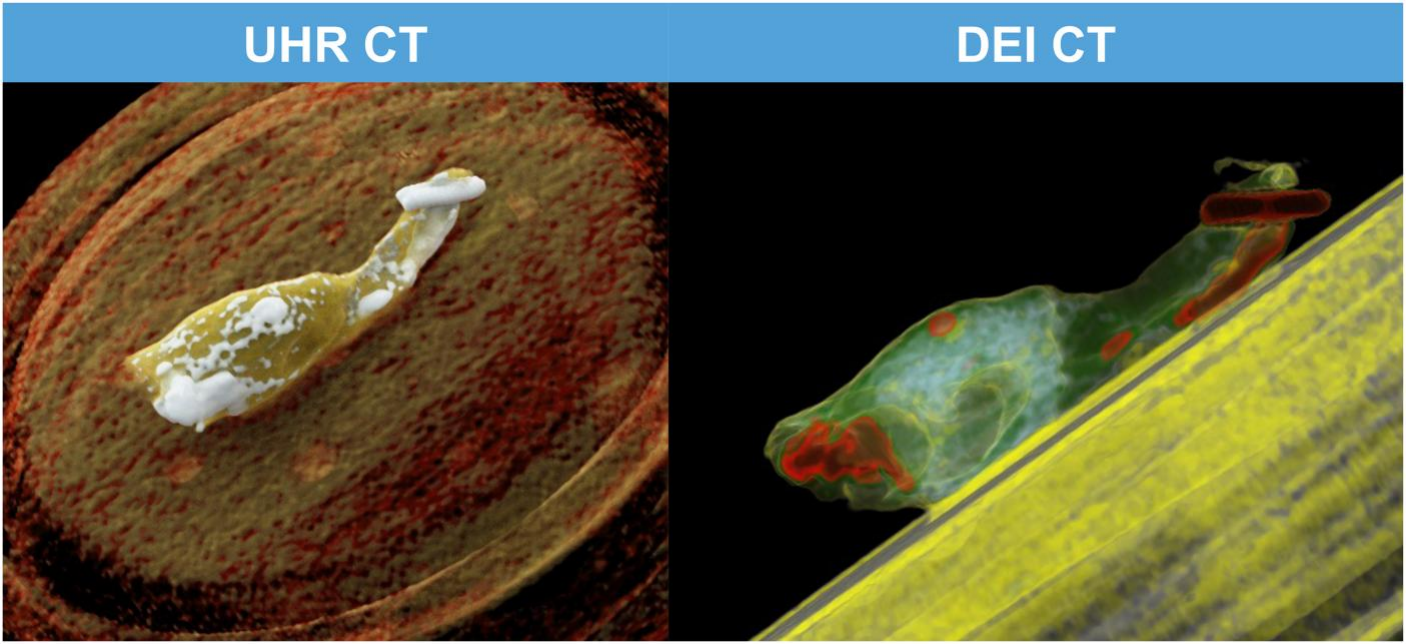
3D ultra-high-resolution computed tomography and dual energy index images
